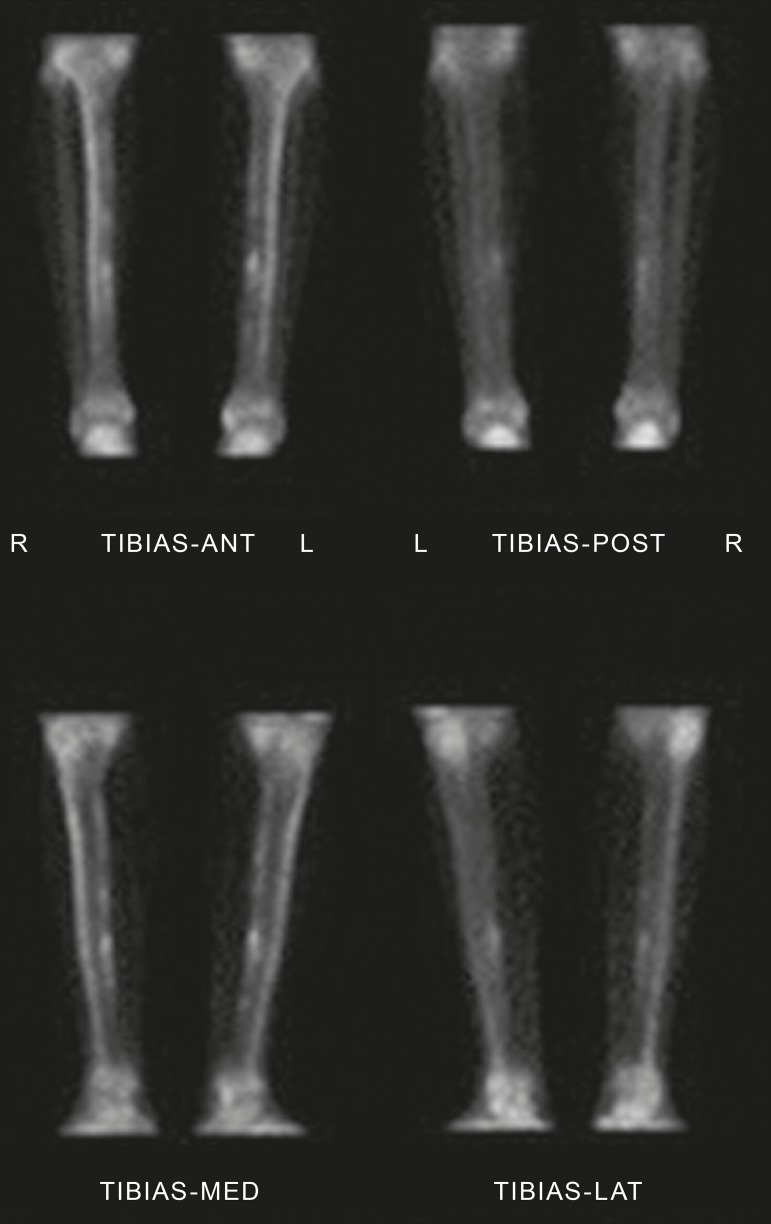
Quantitative analysis of bone scintigraphy.
